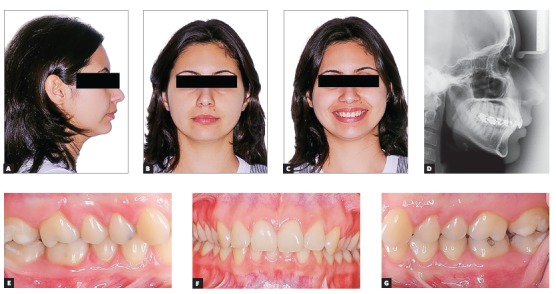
Initial photographs: extraoral (A, B, C), radiograph (D) and intraoral (E, F, G).
